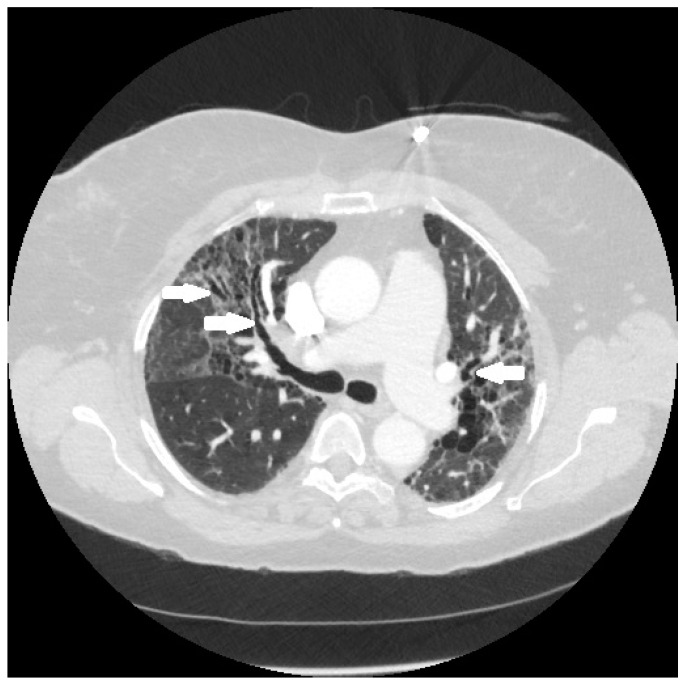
Chest CT scan of fibrotic pulmonary sarcoidosis. The fibrosis is typically in a peribronchial location, which in this case has resulted in fibrosis-induced traction bronchiectasis of airways (arrows).
